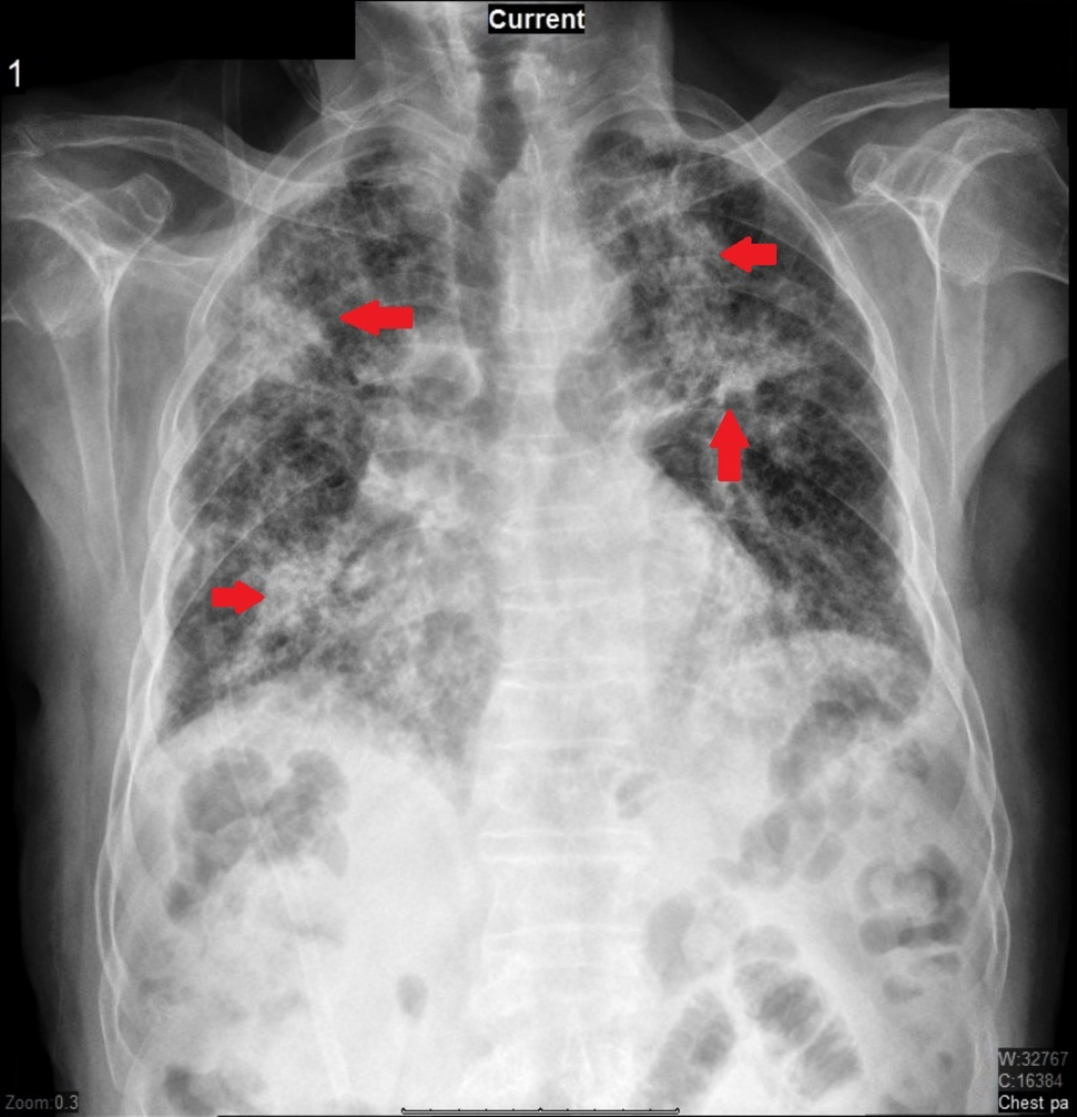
Chest radiography showing increased reticular markings and areas of consolidation suggestive of multilobular pneumonia
